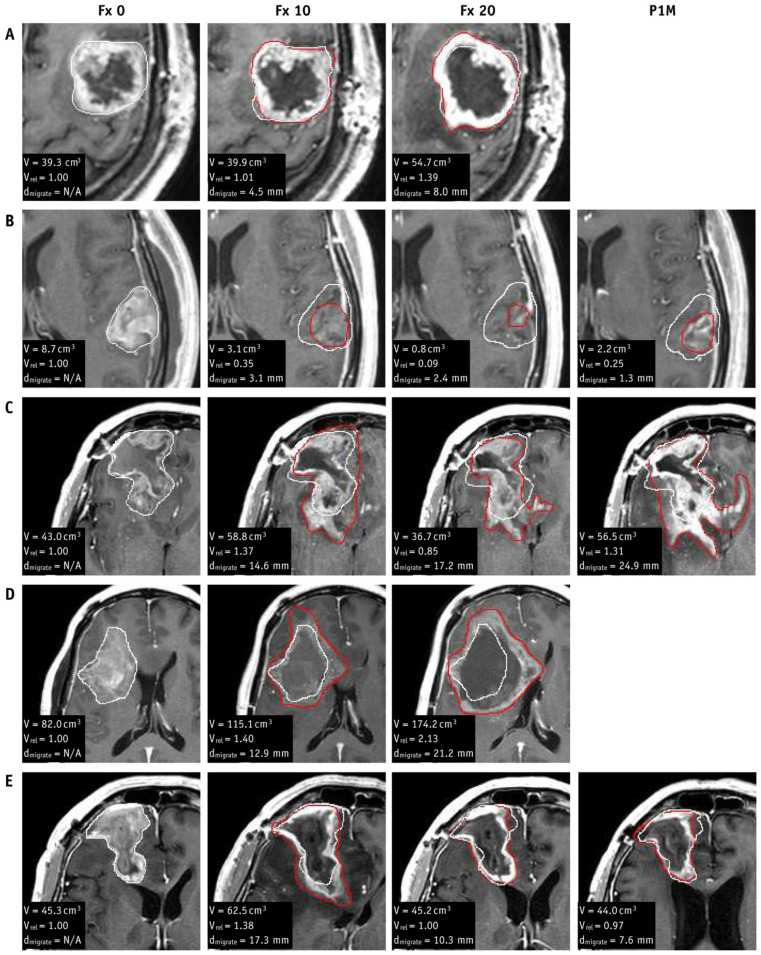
Glioblastomas can change rapidly during treatment. Zoomed images of contrast-enhanced T_1_-weighted MRI for five cases of glioblastomas (A–E) at treatment planning (Fx 0), fractions 10 and 20 (Fx 10 and Fx 20), and one-month post-radiotherapy (P1M). The white contour shows the gross tumor volume (GTV) at planning while the red contour shows the GTV at the specified timepoint. The absolute volume (V), the volume relative to planning (V_rel_), and the maximum distance of the new GTV from the planning GTV (d_migrate_) are listed in the lower left-hand corner of each image. Figure was reproduced from Stewart et al. with permission from the publisher [26].
